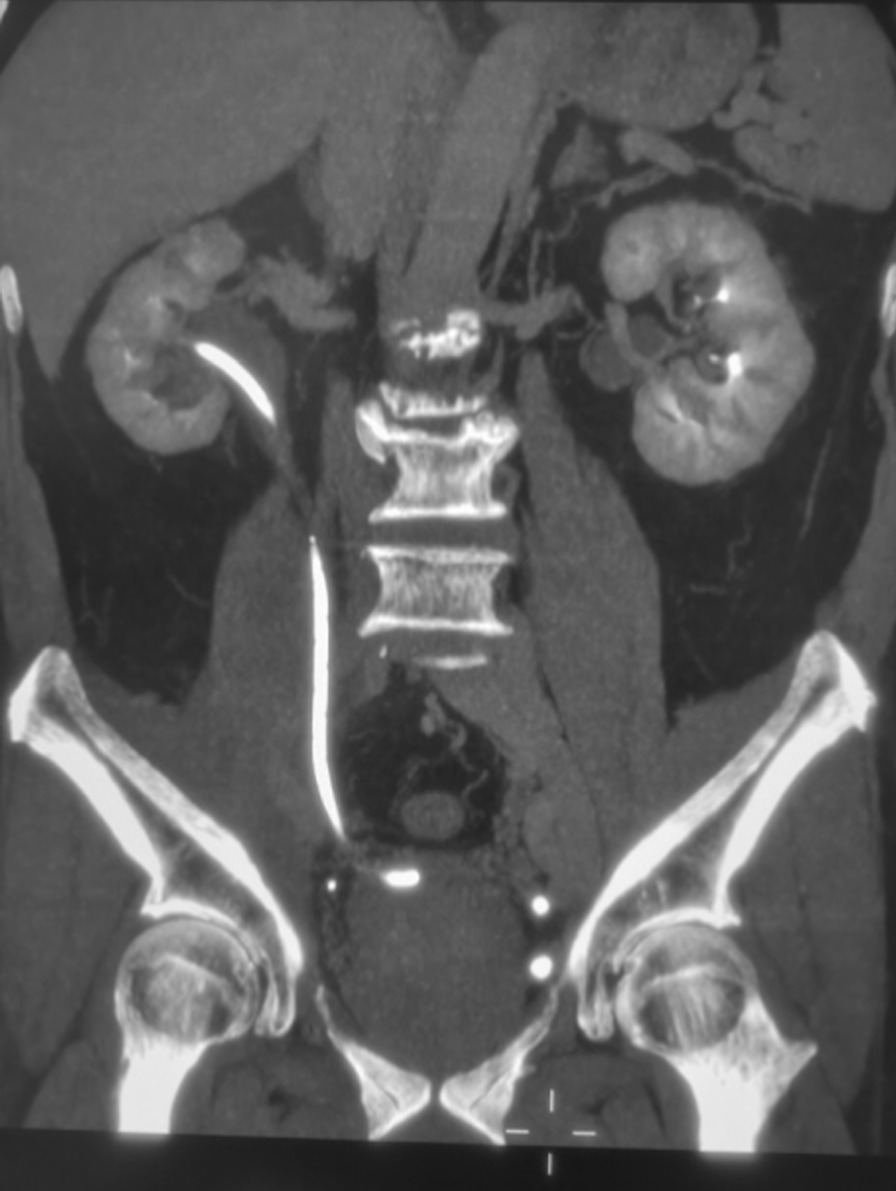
Recheck CT to confirm that the position of drainage tube is satisfactory
